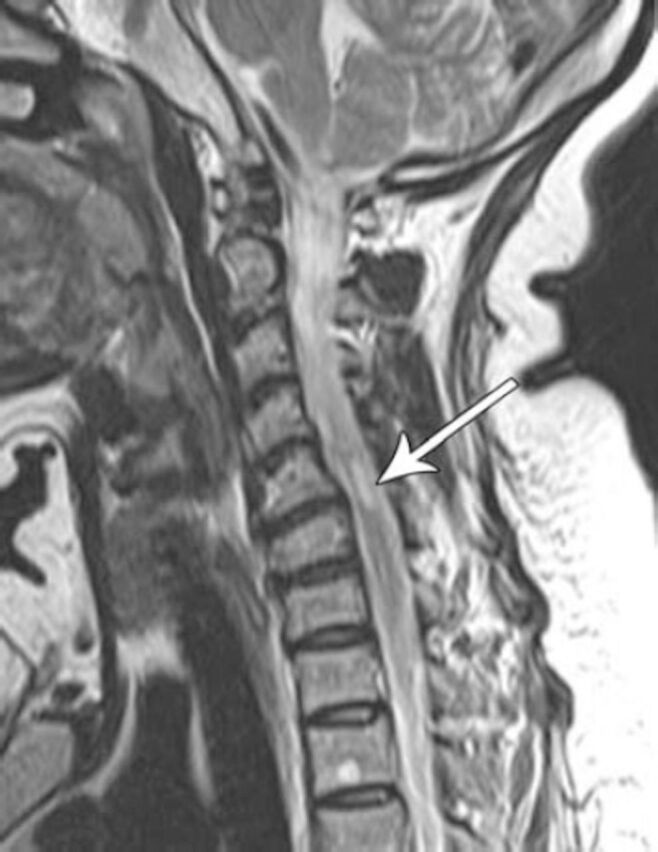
Sagittal T2 MRI of the cervical spine of a 38-year-old female who underwent an epidural steroid injection of the neck showing a right-sided intramedullary area of abnormal signal at the C5-6 level.
